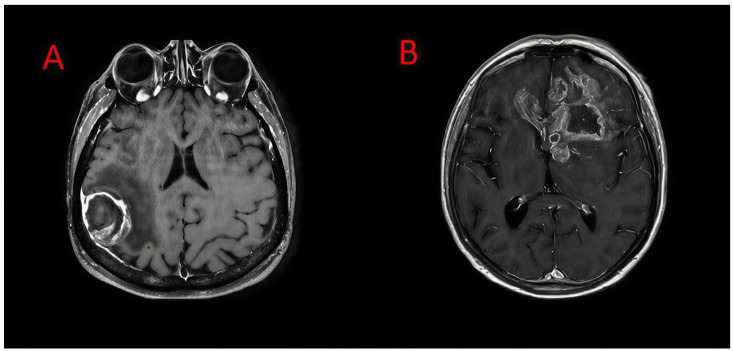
Representative axial contrast-enhanced T1-weighted MRI images demonstrating different levels of tumor burden. (A) Lower tumor burden with relatively limited contrast-enhancing lesion. (B) Higher tumor burden with more extensive contrast enhancement, associated with deeper structural involvement, contralateral extension, and mass effect.
